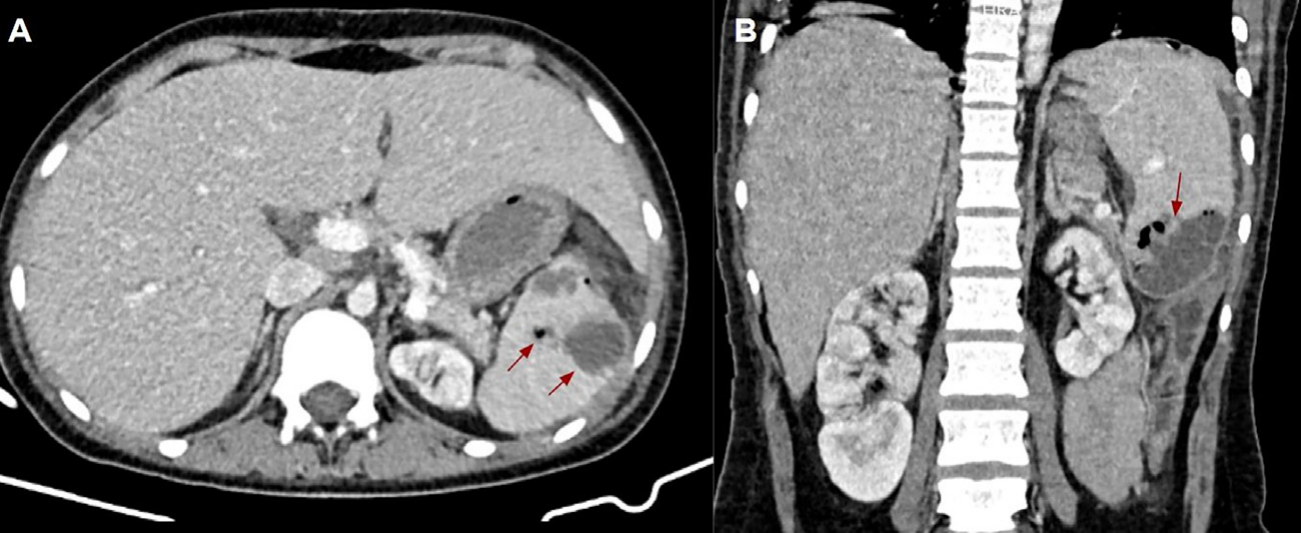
(A and B) Abdominal CT scan with intravenous contrast material demonstrates confluent hypodense collections containing air bubbles (red arrows) in the lower pole of the spleen which is compatible with the diagnosis of splenic abscess in axial and coronal view, respectively.
